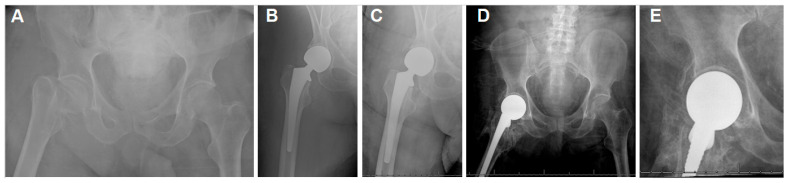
(A) The plain hip radiographs of a 68-year-old man who presented with a displaced right femoral neck fracture. (B) Postoperative plain hip radiograph taken immediately after cemented bipolar hemiarthroplasty. (C) Two-week postoperative plain pelvic radiograph showing stable HA and early HO. (D,E) Seventeen-month postoperative plain hip AP radiographs showing class 4 HO.
